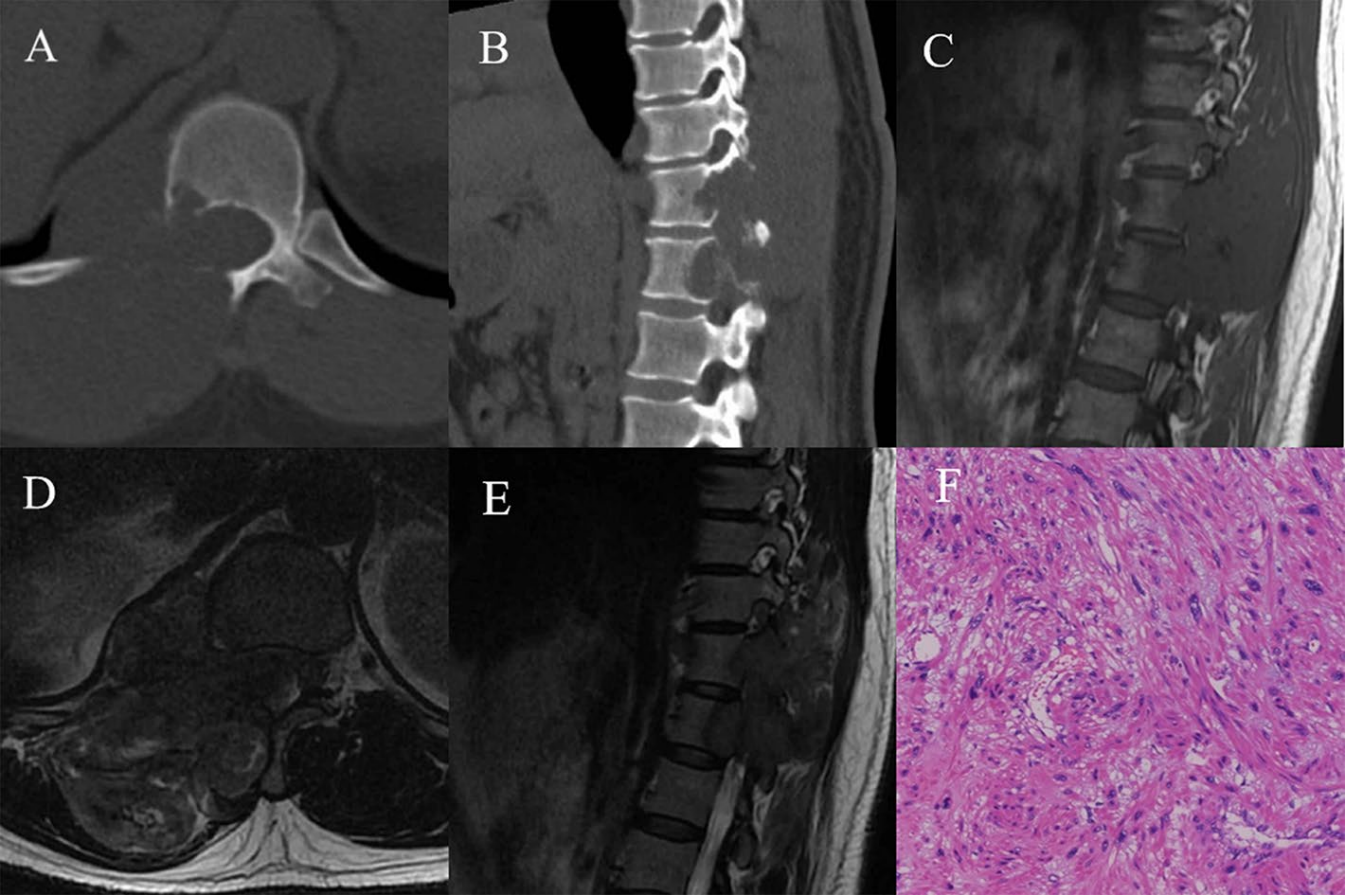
A 44-year-old female with T11–T12 primary leiomyosarcoma. A Axial computed tomography (CT) bone window showed that the mass destroyed the surrounding bone; B Sagittal CT bone window showed bone destruction of T11–T12 vertebral body and posterior elements, with residual bone trabeculae; C T1 weighted imaging (T1WI) in sagittal view showed an isointense mass; D T2 weighted imaging (T2WI) in axial view showed a lobulated mass with ill-defined border; E T2WI in sagittal view showed an isointense mass; F The interstitial tumor showed diffuse spindle cell hyperplasia with many nuclear fissions (HE; ×400)
